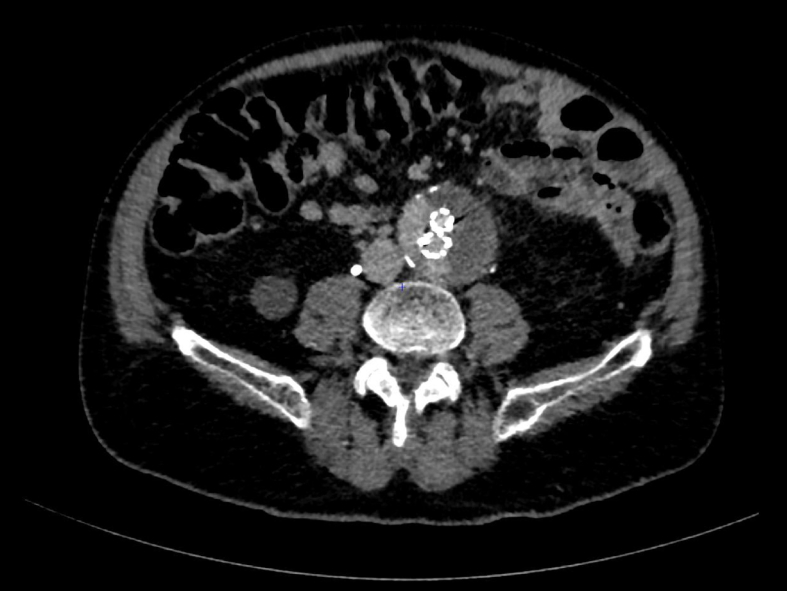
CT scan with contrast, axial view: aneurysmal sac opacification in the right side of the aortic graft.
